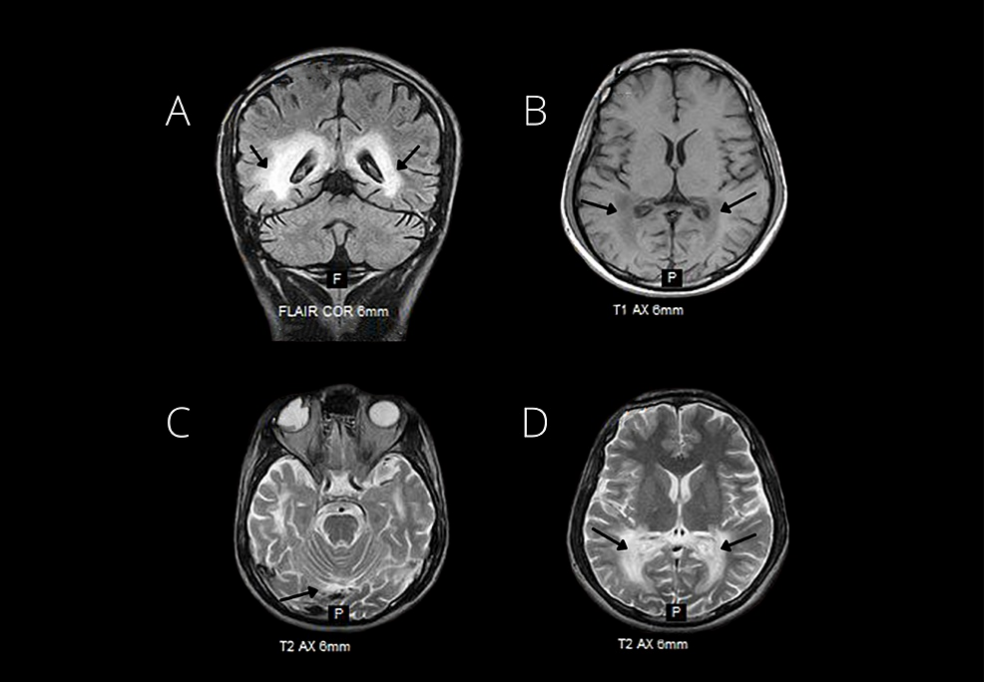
MRI brain scan of a 20-year-old male with a history of Addison's disease at the time of suspected X-ALD This shows bilateral symmetrical confluent areas of signal abnormalities in parieto-occipital, temporal lobe, and splenium of the corpus callosum. (A) Fluid-attenuated inversion recovery (FLAIR), showing hyperintensity or high signals in marked areas. (B) T1-weighted image shows hypointensity or low signals in the marked areas. (C) and (D) T2-weighted images also show hyperintensity or high signals in the marked areas. X-ALD - X-linked adrenoleukodystrophy
